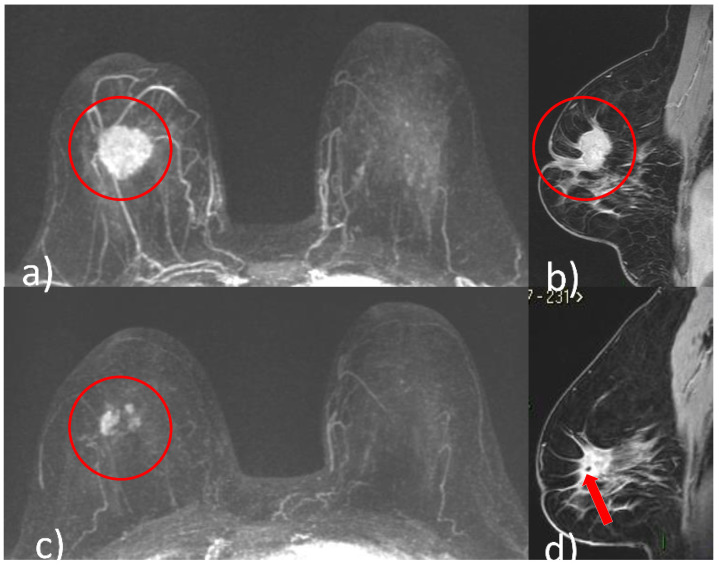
A 48-year-old patient with G2, luminal B, HER2 positive right breast cancer undergoing NACT. Pre-treatment breast CE-MRI showed an oval mass with irregular margins, nipple invasion and skin retraction at the junction of upper quadrants of the right breast (red circle, (a) axial maximum intensity projection reconstruction image) (red circle, (b) sagittal post-contrast T1-weighted image). After NACT, multifocal shrinkage was depicted by CE-MRI (red circle (c), axial maximum intensity projection reconstruction image). (d) Signal void artifact caused by tissue marker clip inside the residual mass is well visible on the sagittal T1-weighted post-contrast image (red arrow).
